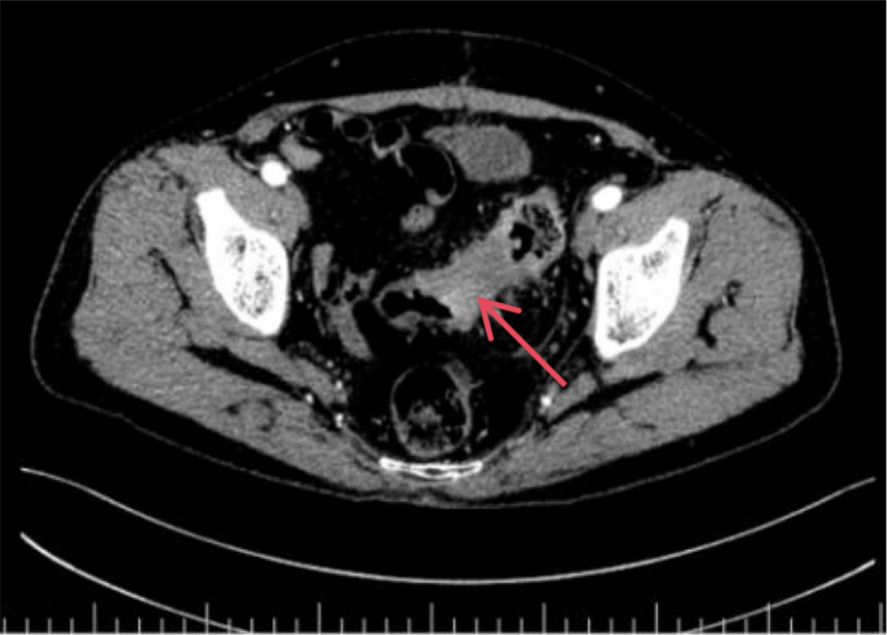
Enhanced CT of the lower abdomen and pelvis: The sigmoid colon wall appears rigid with marked heterogeneous thickening. The lumen shows irregular narrowing. The inner margin of the lesion is irregularly indented, while the outer margin is rough. After contrast enhancement, the affected bowel wall demonstrates markedly heterogeneous enhancement. The peritoneal fat space appears hazy. Multiple soft tissue density nodular shadows are visible around the bowel, with the largest measuring approximately 1.1 × 1.0 cm. (The red arrow in the image points to the affected area.).
